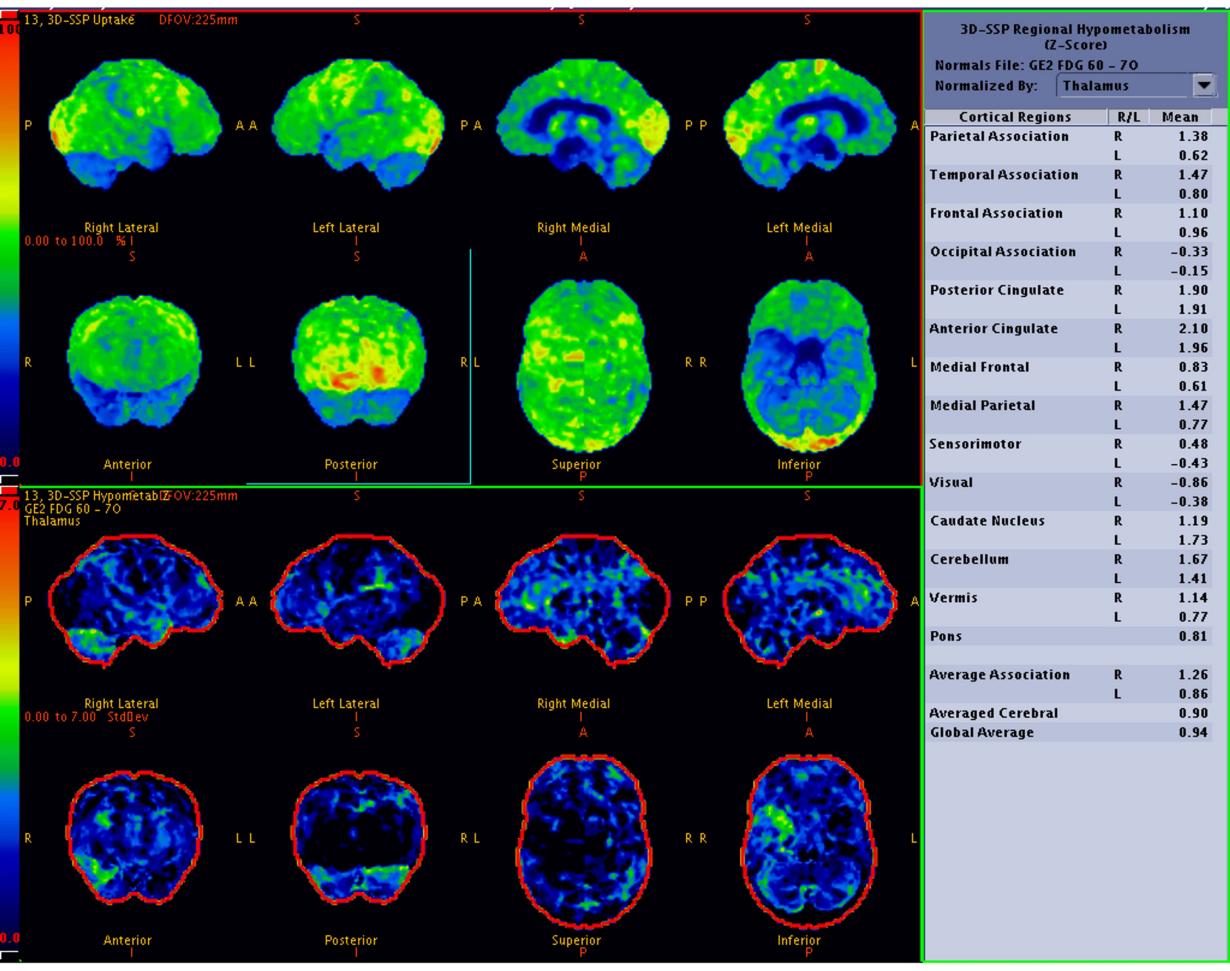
Brain FDG PET-CT scan of a 64-year-old man with carcinoma of the left lung and an eight-year history of diabetes mellitus, on oral hypoglycemic agents (FBS: 126 mg/dL; MMSE score: 21), showing global hypometabolism with sparing of the sensorimotor cortex and reduced uptake in the posterior cingulate gyrus and precuneus, features suggestive of an Alzheimer's disease-like pattern. The upper two rows show the Z-score maps of cerebral hypometabolism, while the lower two rows represent the inverse images FDG PET-CT: fluorodeoxyglucose positron emission tomography-computed tomography; FBS: fasting blood sugar; MMSE: Mini-Mental State Examination; 3D-SSP: three-dimensional stereotactic surface projection; R: right; L: left; S: superior; I: inferior; A: anterior; P: posterior
